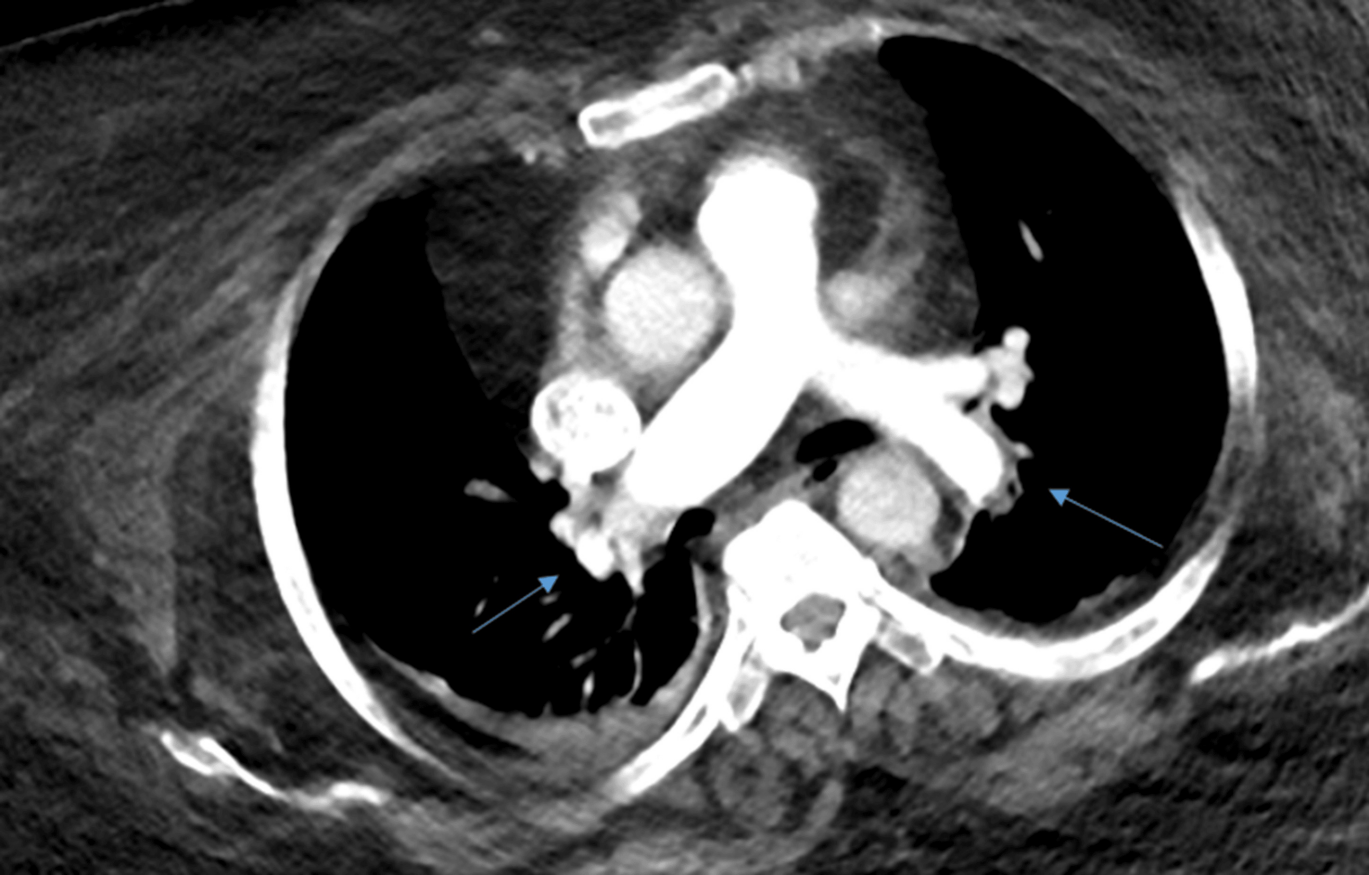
CTPA showing the resolution of pulmonary embolism with blue arrows indicating areas of reperfusion after ultrasound-assisted catheter-directed thrombolysis CTPA: computed tomography pulmonary angiography
